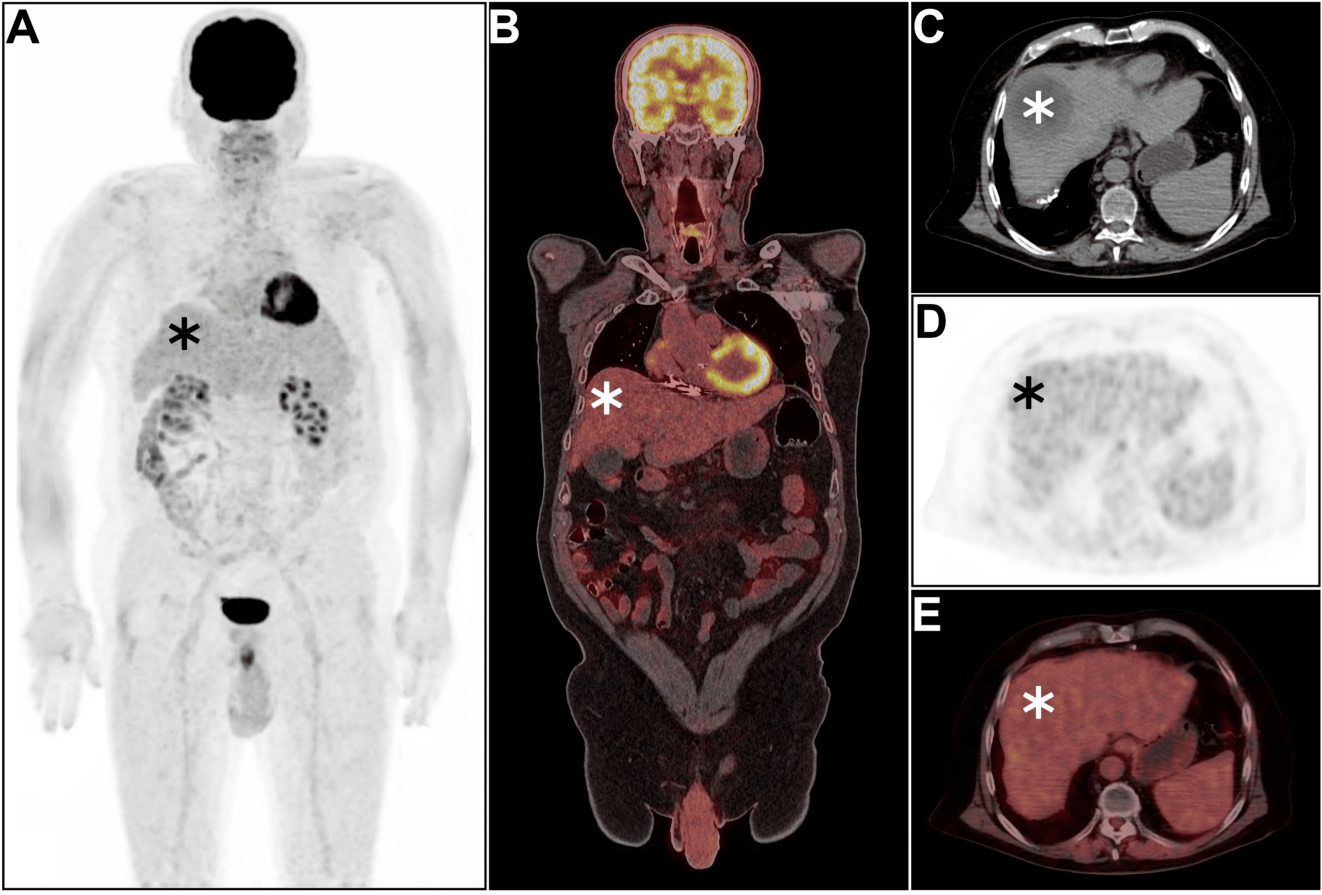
Baseline [18F]FDG PET/CT (A-E) Maximum intensity projection [^18^F]FDG image, fused coronal, and axial PET/CT views depicted the hepatocellular carcinoma lesion as an isometabolic segment VII hypodensity with otherwise unmarkable scan elsewhere (asterisks). FDG: Fluorodeoxyglucose
